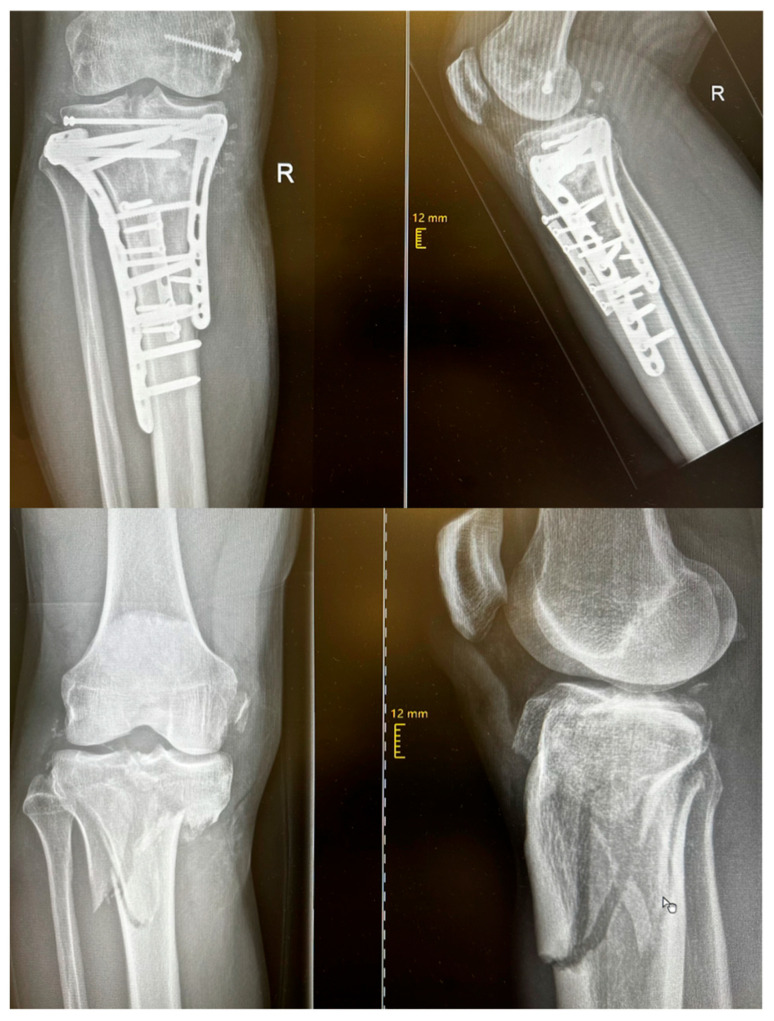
Radiographs of a 44-year-old patient with a tibial plateau fracture sustained during skiing. Following resolution of soft-tissue swelling, surgical treatment was performed via an anterolateral and a posteromedial approach. In addition, a bony avulsion of the medial collateral ligament (MCL) was refixed using screw osteosynthesis.
